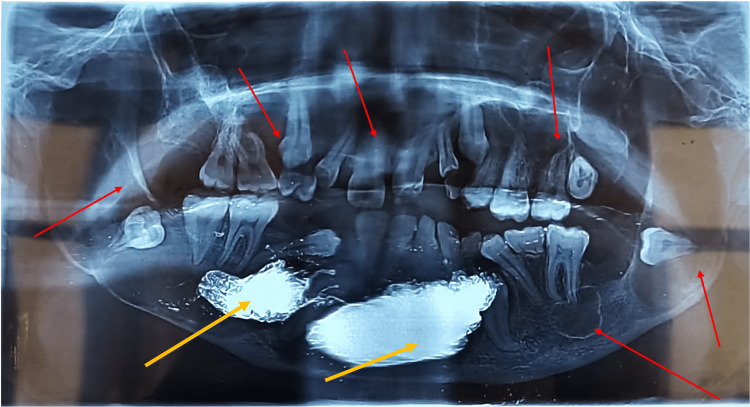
Yellow arrows in orthopantomogram showing two sites of marsupialized area and red arrows showing multiple cysts both in the maxilla and mandible
